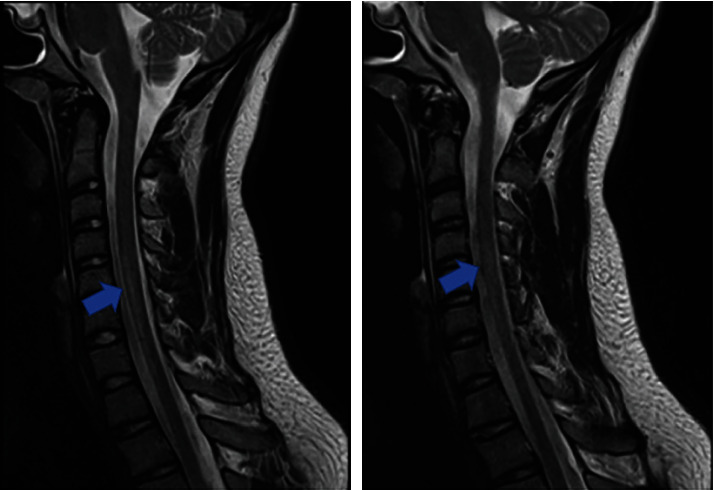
Spine MRI showing an extensive multisegmental T2-weighted hyperintense signal between the median plane of C4 and the transition from C7 to D1 (arrows) with symmetrical anterior medullary involvement and restricted diffusion on DWI, but without spinal cord expansion or signs of spinal cord compression. There was a normal T2 vertebral artery flow void, with no apparent signs of acute vertebral artery dissection or intramural hematoma.
